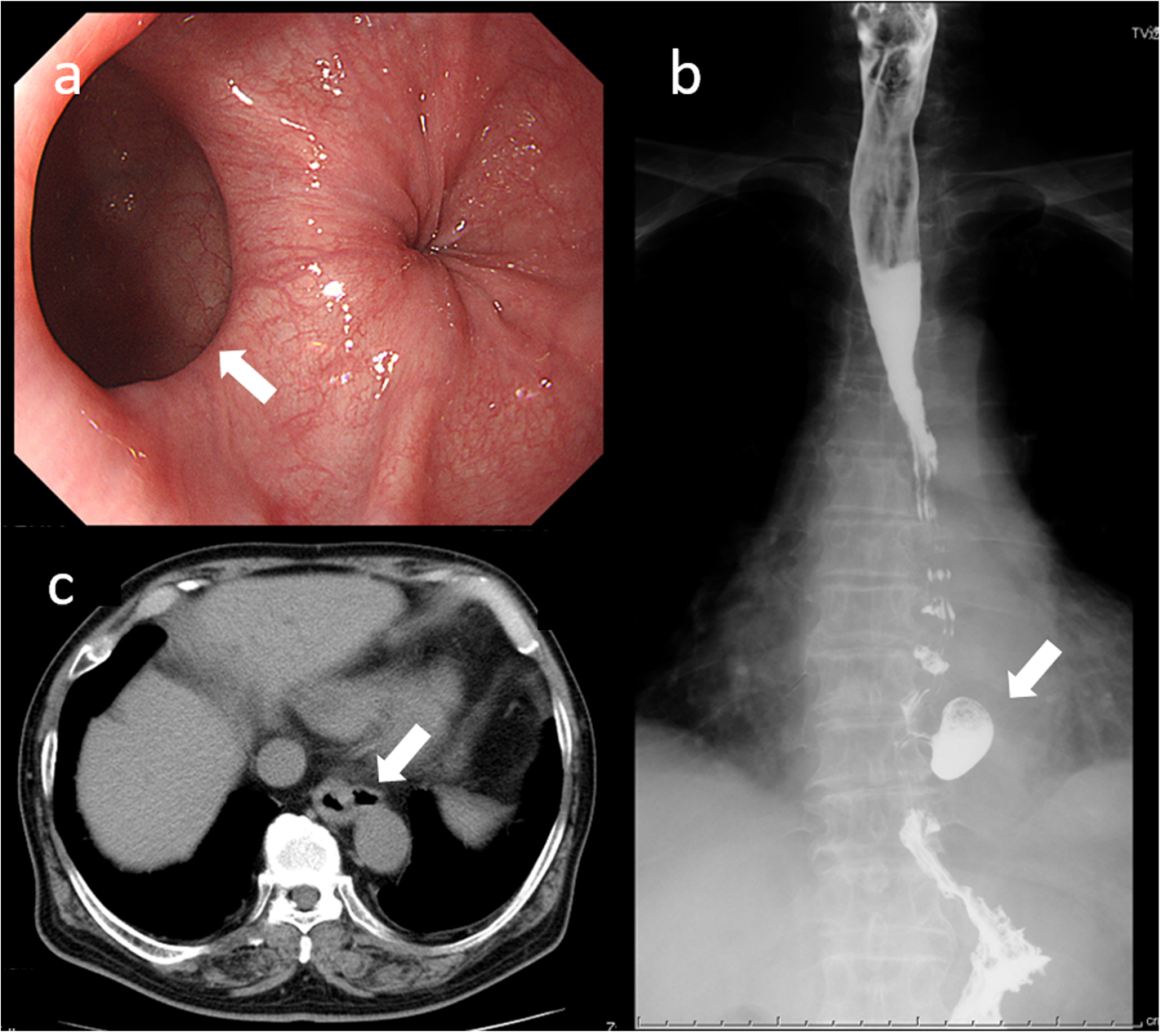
a Upper gastrointestinal endoscopy showed a 31-mm diverticulum on the left wall of the lower esophagus (arrow). b A barium esophagogram showed a diverticulum in the lower esophagus 50 mm above the EGJ (arrow). c Chest CT revealed a diverticulum on the left wall in the lower esophagus with no esophageal masses (arrow)
